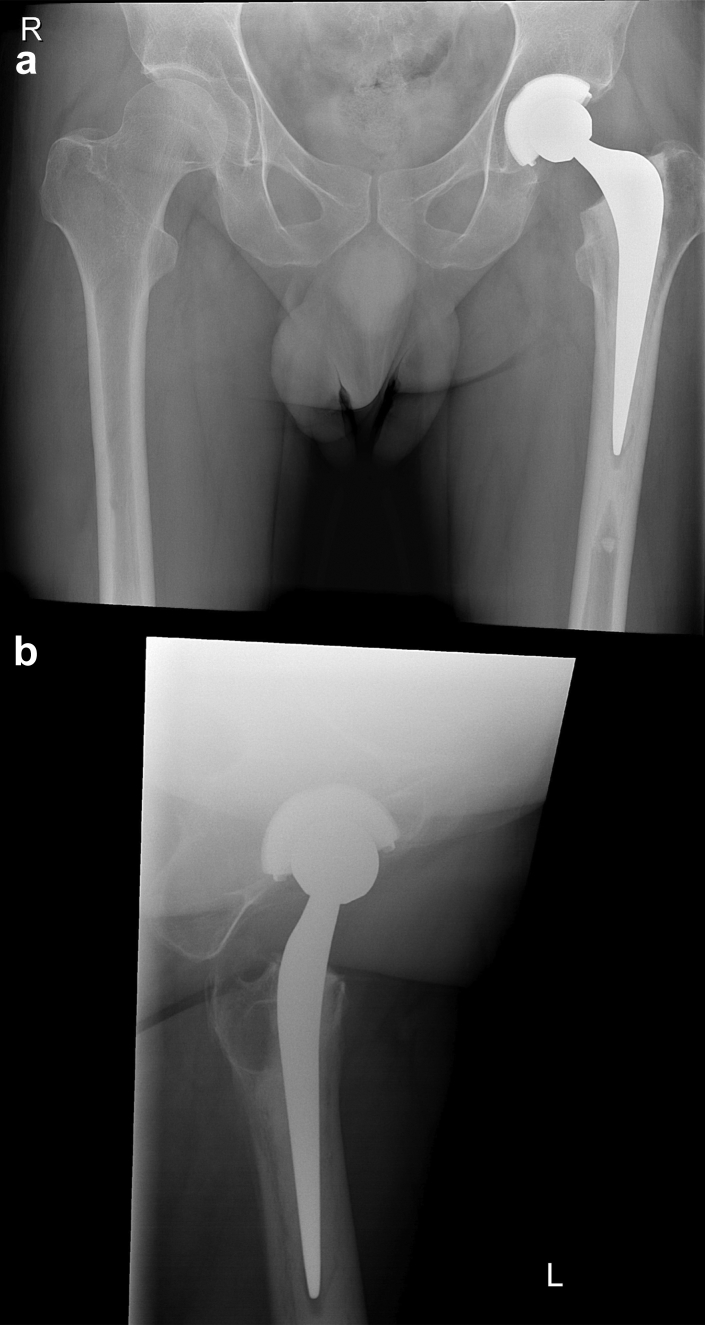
Plain anteroposterior (a) pelvic and lateral (b) hip radiographs of the index surgery showing Barack type A cementing “white out” mantle with a central position of the prosthesis in both planes.
